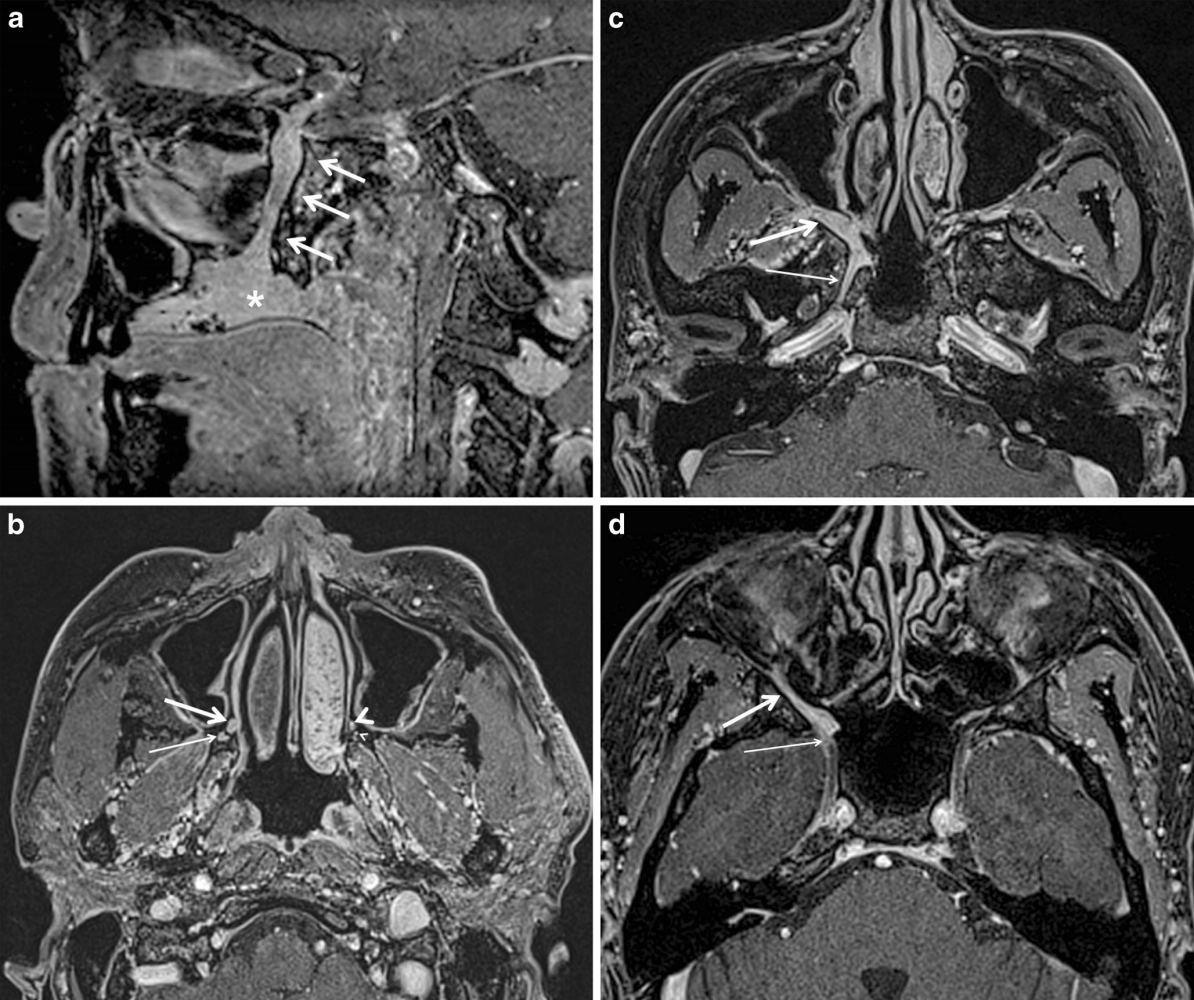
a ACC of the hard palate in a 33-year-old female patient. Sagittal contrast-enhanced FS T1W MR image shows an enhancing mass involving the hard palate (*asterisk*) with PNS along the right greater palatine nerve (*arrows*). b Axial contrast-enhanced FS T1W MR image in the same patient obtained at the level of the inferior apex of the PFF shows the enlarged, enhancing right greater palatine nerve (*thick arrow*) and lesser palatine nerve (*thin arrow*) due to PNS. Note the normal left greater palatine nerve (*thick arrow head*) and lesser palatine nerve (*thin arrow head*). c Axial contrast-enhanced FS T1W MR image in the same patient at a higher level shows disease spread from the right PPF (*thick arrow*) into the right Vidian canal (*thin arrow*). d Axial contrast-enhanced FS T1W MR image in the same patient obtained at the level of the FR (*thin arrow*). It shows a thickened and abnormally enhancing right ION (*thick arrow*) due to PNS
